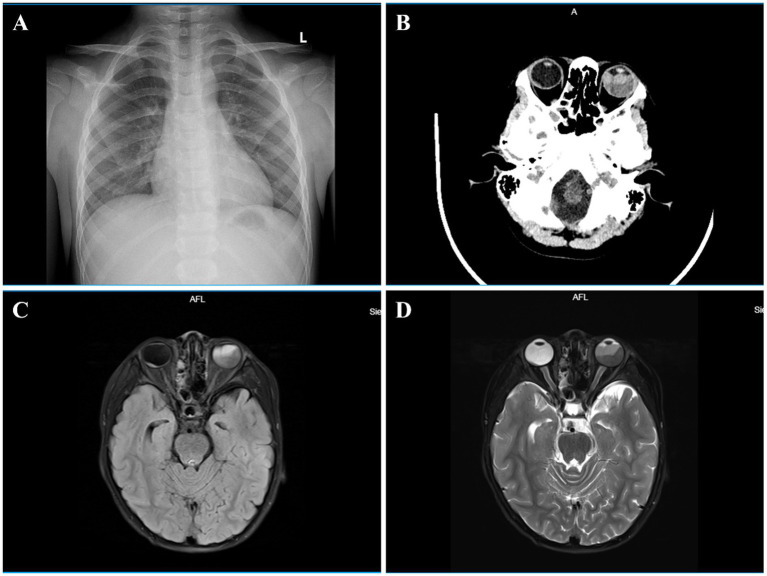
Chest X-ray* (anteroposterior view) revealed no cardiopulmonary abnormalities in the pediatric patient (A). *Cranial CT* showed a heterogeneous-density lesion (maximal cross-section 1.8 × 2.7 cm) within the left eyeball with inhomogeneous contrast enhancement and mild morphological distortion, suggestive of neoplastic lesions (B). *Cranial MRI* further revealed morphological distortion of the left eyeball containing a nodular mixed-signal lesion (maximal transverse section 2.3 × 1.8 cm), exhibiting slightly hyperintense signal on T1WI, layered hypointense to mildly hypointense signal on T2WI, corresponding to hypointense or slightly hyperintense signal on FLAIR, with post-contrast heterogeneous enhancement in T2WI-hypointense areas and no significant enhancement in T2WI-mildly hypointense regions. Additionally, mild thickening and enhancement were noted in the posterior wall of the right eyeball (C,D).
